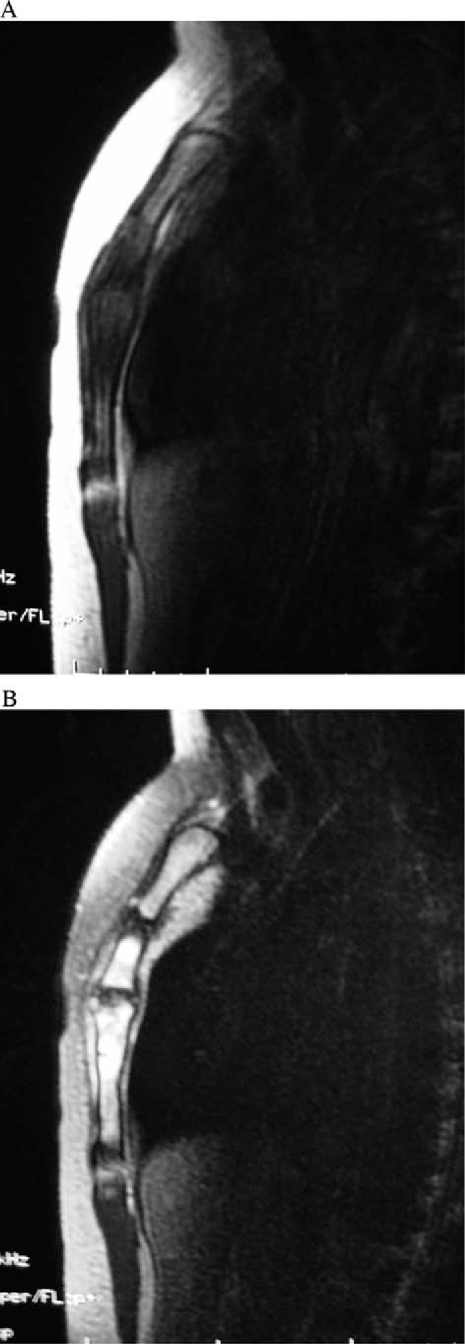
Magnetic resonance imaging (MRI). The signal intensity of the tumor was low in the sternum on T1-weighted images (A) and high on T2-weighted images (B).
